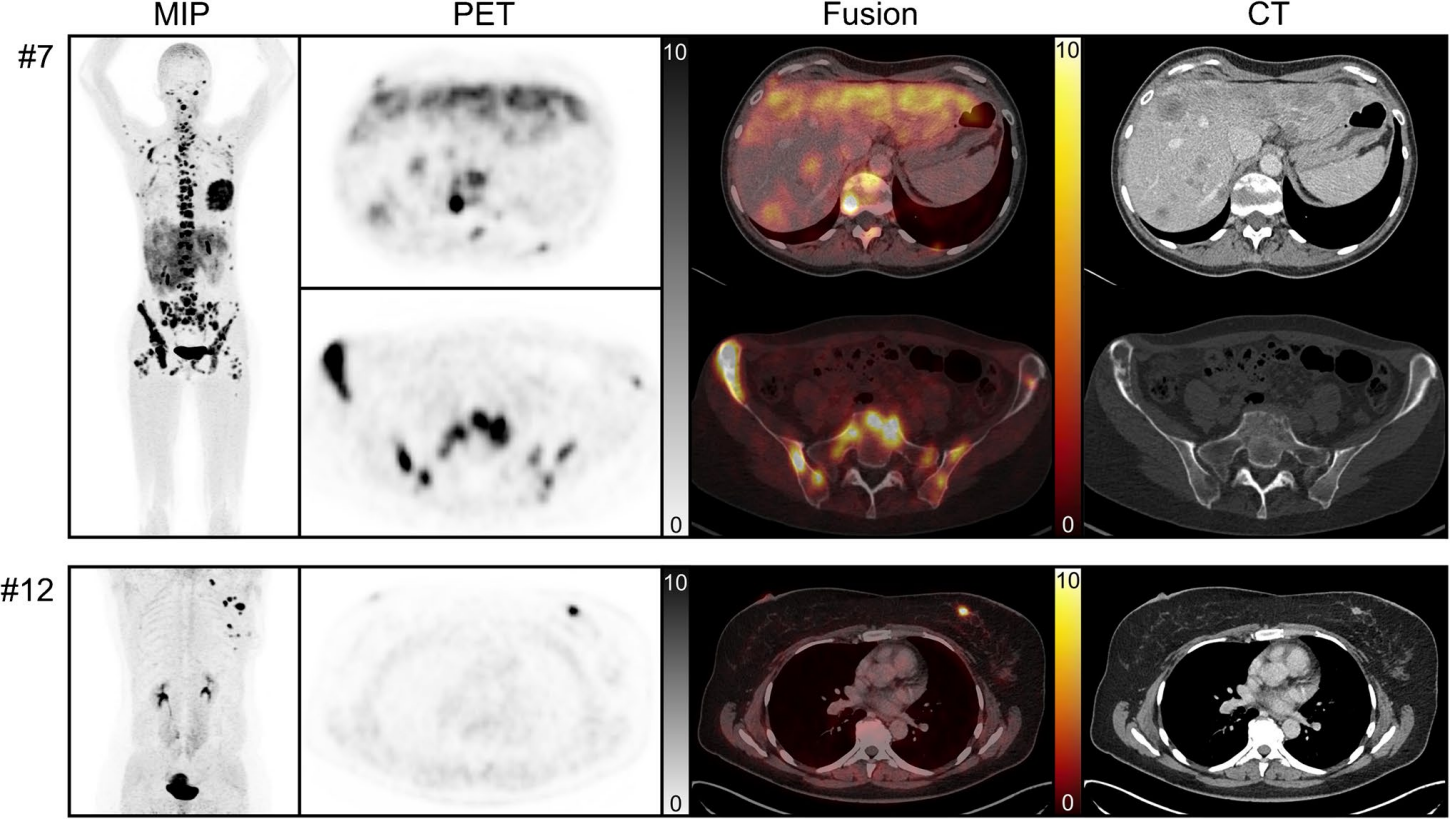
Two examples of patients scanned with ^68^Ga-OncoFAP. Patient #7 with newly widespread metastatic disease to lymph nodes, bones, and liver at primary diagnosis of locally advanced breast cancer. Whole-body maximum intensity projection (MIP), PET, fusion, and contrast-enhanced CT are shown in a slice at the liver and the pelvis demonstrating osseous (SUV_max_ 19.5) and hepatic metastases (SUV_max_ 9.9). Patient #12 with newly diagnosed breast cancer metastatic to local and supraclavicular lymph nodes. MIP, PET, fusion, and contrast-enhanced CT are shown in a slice at the breast demonstrating strong uptake of even sub-centimeter breast lesions (SUV_max_ 15.1). All PET images are adjusted to SUV 0–10
